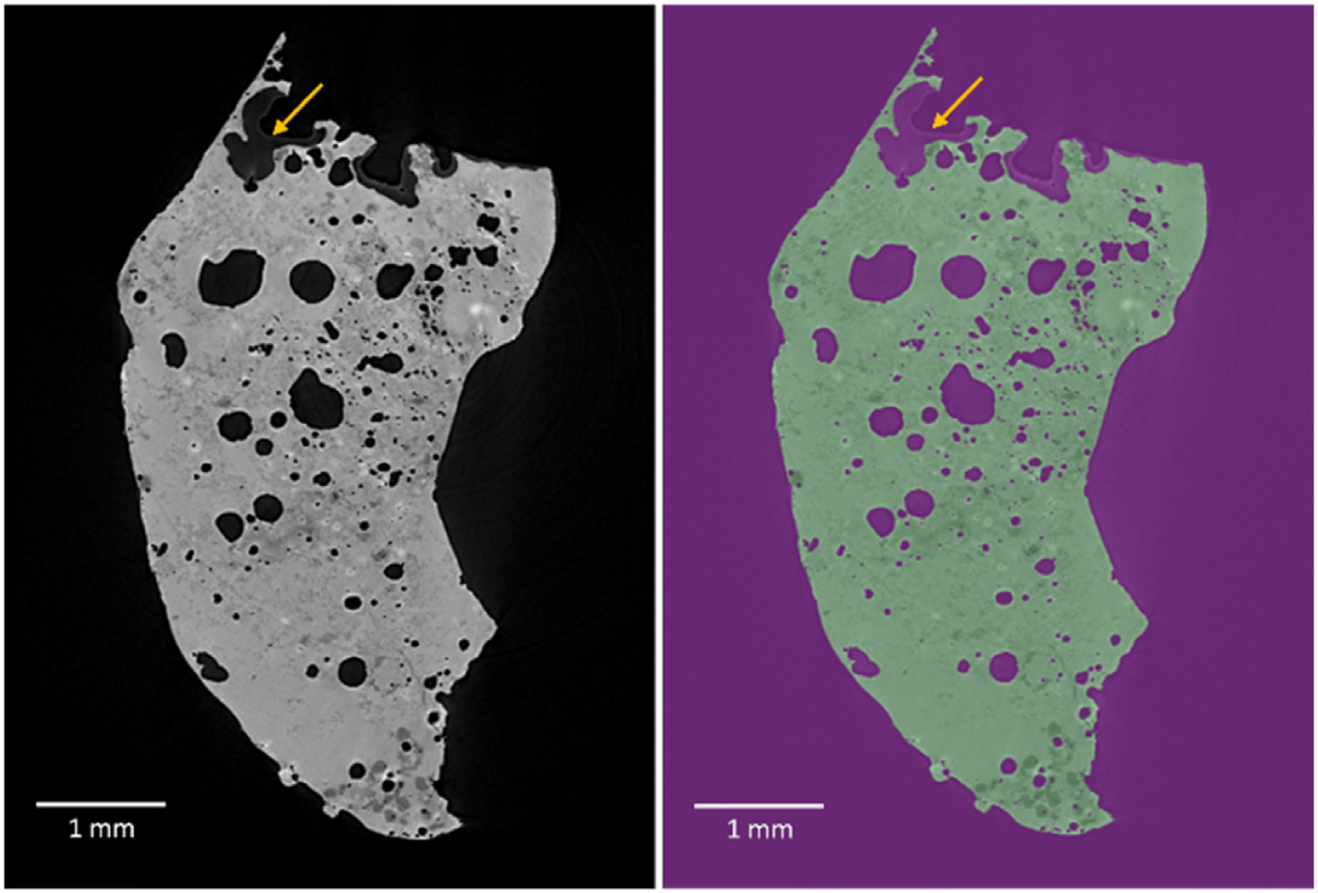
An ortho slice, showing the cross-section of the sample, with the grey scale apply to X-ray attenuation intensities to the left and the labelled one used for model training to the right, where green is the ash and purple the air. In the top of the ash particle, some glue can be seen, indicated with an arrow.
